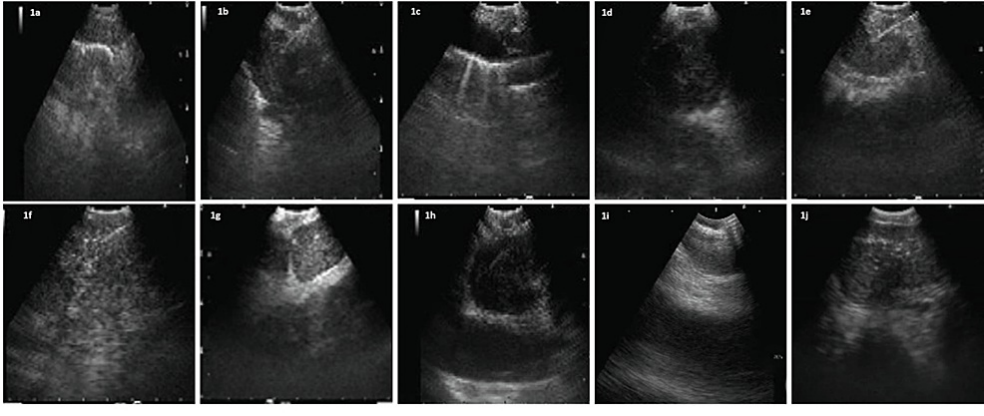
Representative images of endobronchial ultrasound images of lymphnodes. (a) Oval shape; (b) round shape; (c) small size (<10 mm); (d) large size (>10 mm); (e) distinct margins; (f) indistinct margins; (g) homogenous appearance; (h) heterogenous appearance; (i) central hilar structure absent; (j) coagulation necrosis sign present.
